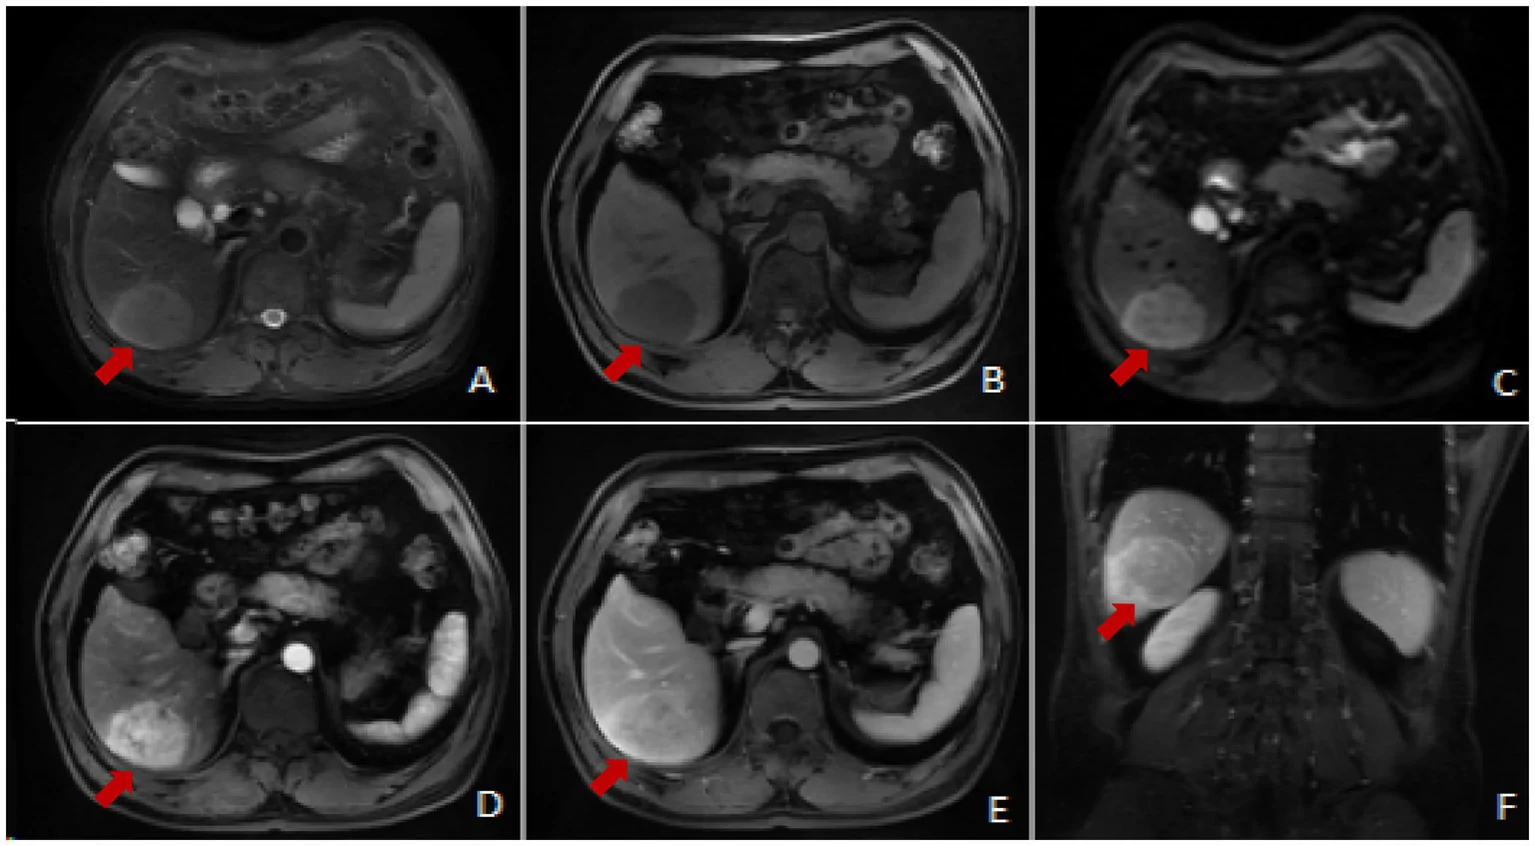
(A) MR T2-weighted imaging (T2WI) shows slightly high signal intensity in the lesion of the right posterior hepatic lobe. (B) T1-weighted imaging (T1WI) shows low signal intensity. (C) Diffusion-weighted imaging (DWI, *b* = 600 s/mm) shows high signal intensity. (D) Arterial phase shows significant enhancement. (E) Portal venous phase shows clear delineation of the lesion. (F) Coronal delayed phase shows ring-like capsule enhancement.
